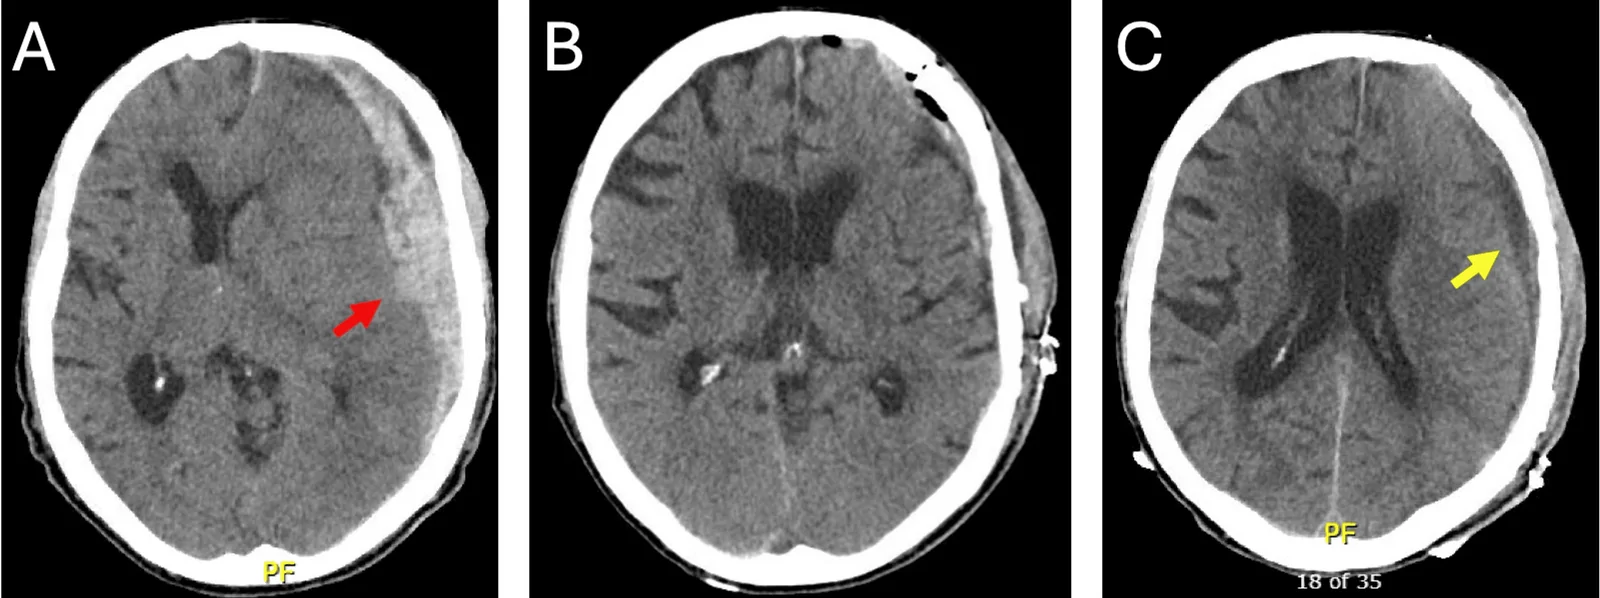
Serial CT imaging of the brain (A) Initial non-contrast CT of the head demonstrating a left-sided subdural hematoma (red arrow) with associated mass effect and midline shift following traumatic injury requiring hemicraniectomy. (B) Follow-up non-contrast CT of the head obtained on postoperative day 2 demonstrating interval evacuation with improvement in mass effect and expected postoperative changes. (C) Repeat non-contrast CT of the head obtained 10 days postoperatively demonstrating interval enlargement of the left subdural collection (yellow arrow) with mass effect confirmed intraoperatively to represent subdural empyema. CT: computed tomography
